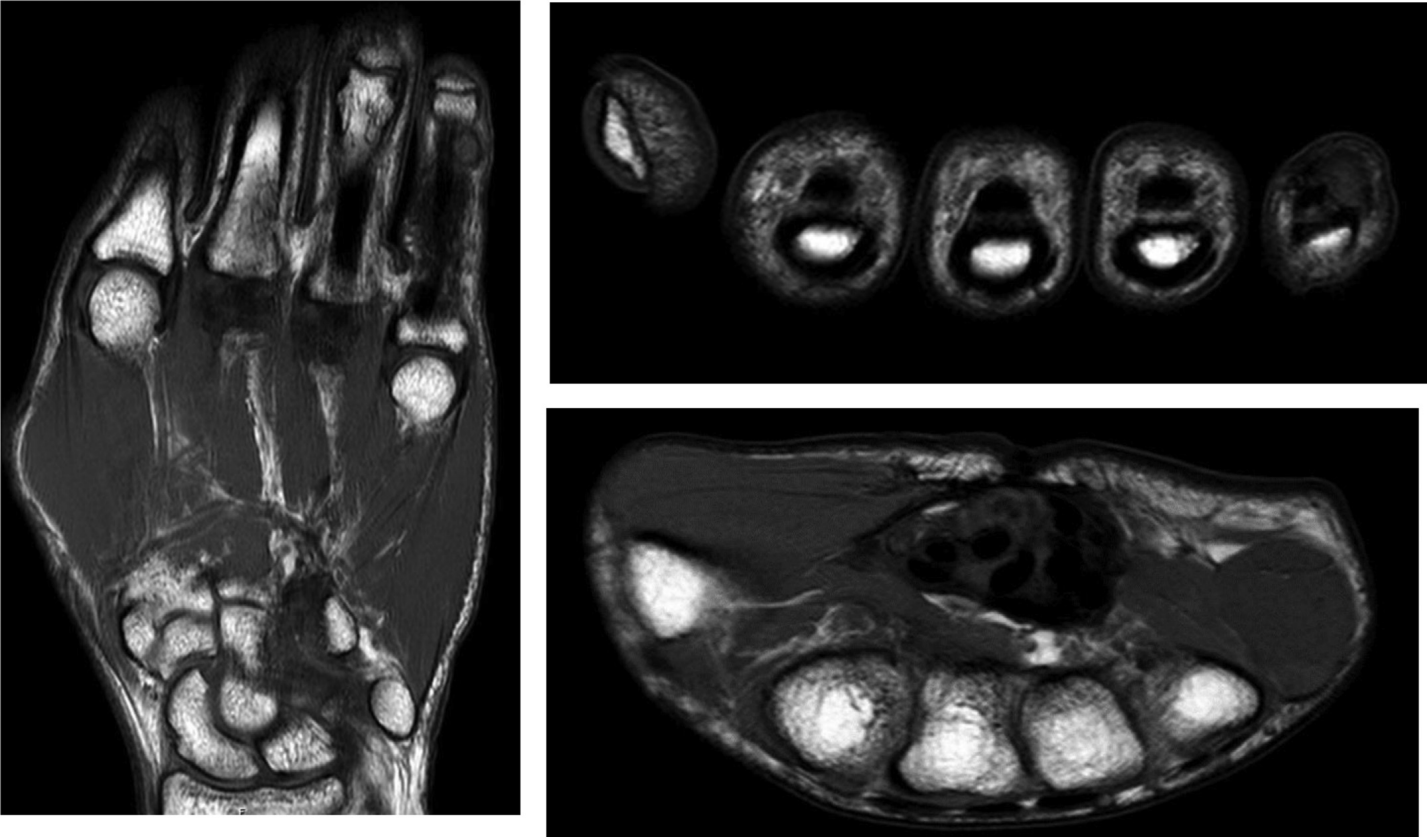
Magnetic resonance imaging findings of second mass recurrence.
